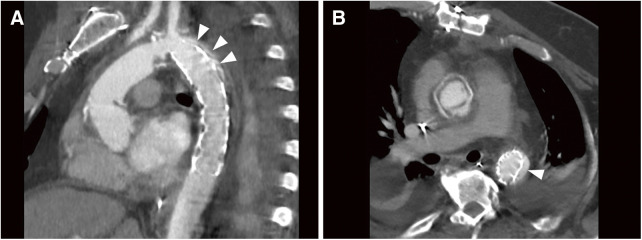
Fig. 1 Enhanced CT imaging (A, B) after the fenestrated FET technique shows antegrade blood flow originating from the fenestration in the FET (arrowheads). The stented portion of the FET shows a circular expansion. CT: computed tomography; FET: frozen elephant trunk
